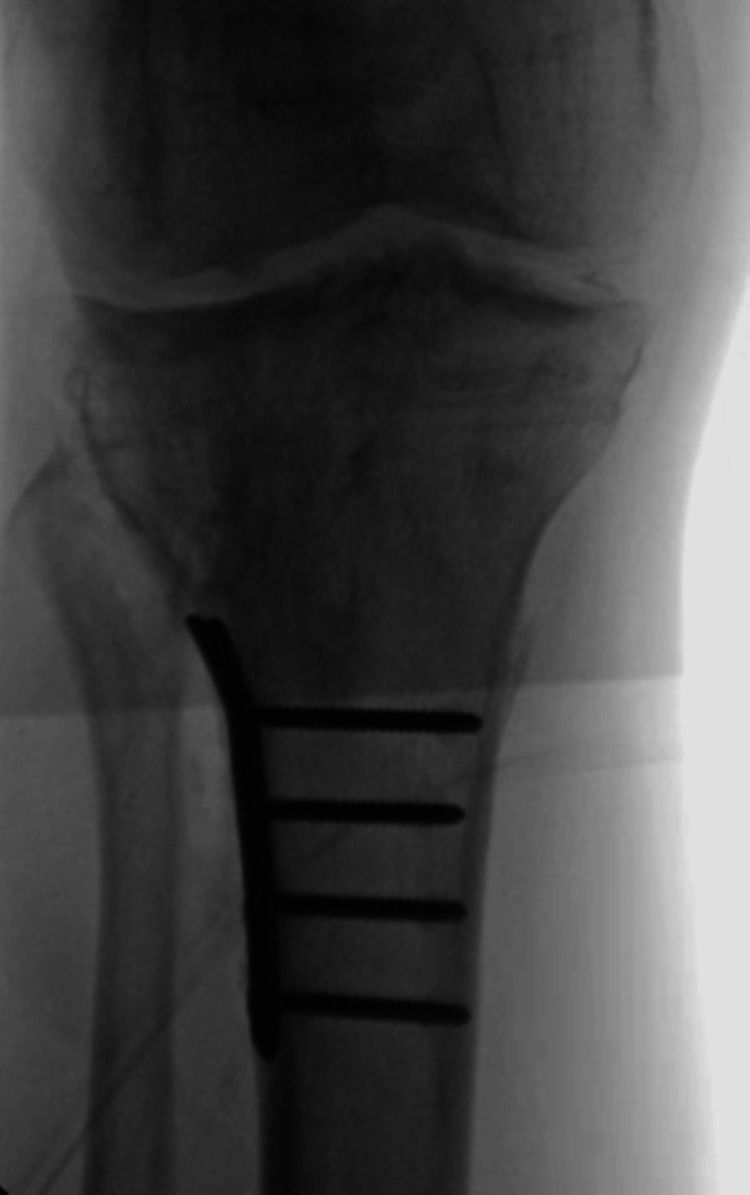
Postoperative radiograph demonstrating removal of proximal periarticular fixation screws with retention of the distal lateral tibial plate segment to preserve cortical stability.
